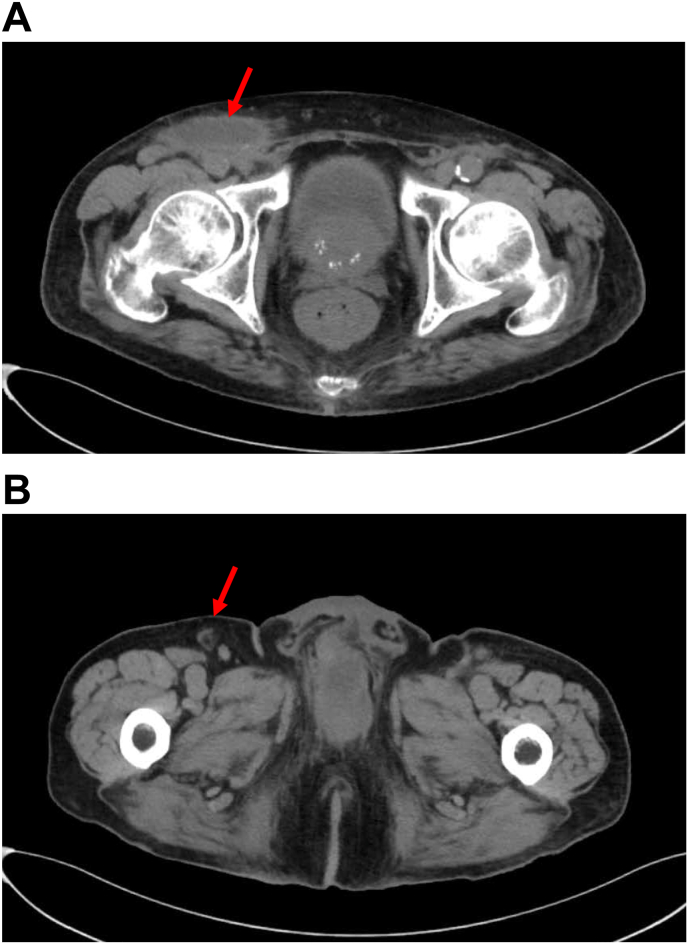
Plain computed tomography taken after right inguinal lymphadenectomy (POD 27) (A) Lymphocele in the right groin (arrow). (B) Residual caudal lymph node measuring 8 mm in the right groin. Lymphangiography was performed via this lymph node (arrow).
